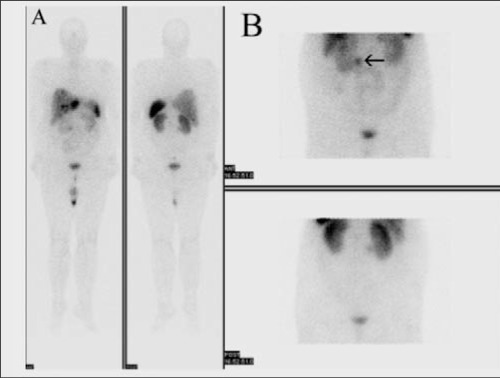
Whole-body (A) and planar (B) somatostatin-receptor scintigraphy at 5 hours show multiple intense focal uptakes of theradiotracer in the liver lesions indicating somatostatin receptor positiveliver metastasis. Planar images (B) show focal tracer uptake at theright side of the upper abdominal region (arrow)
